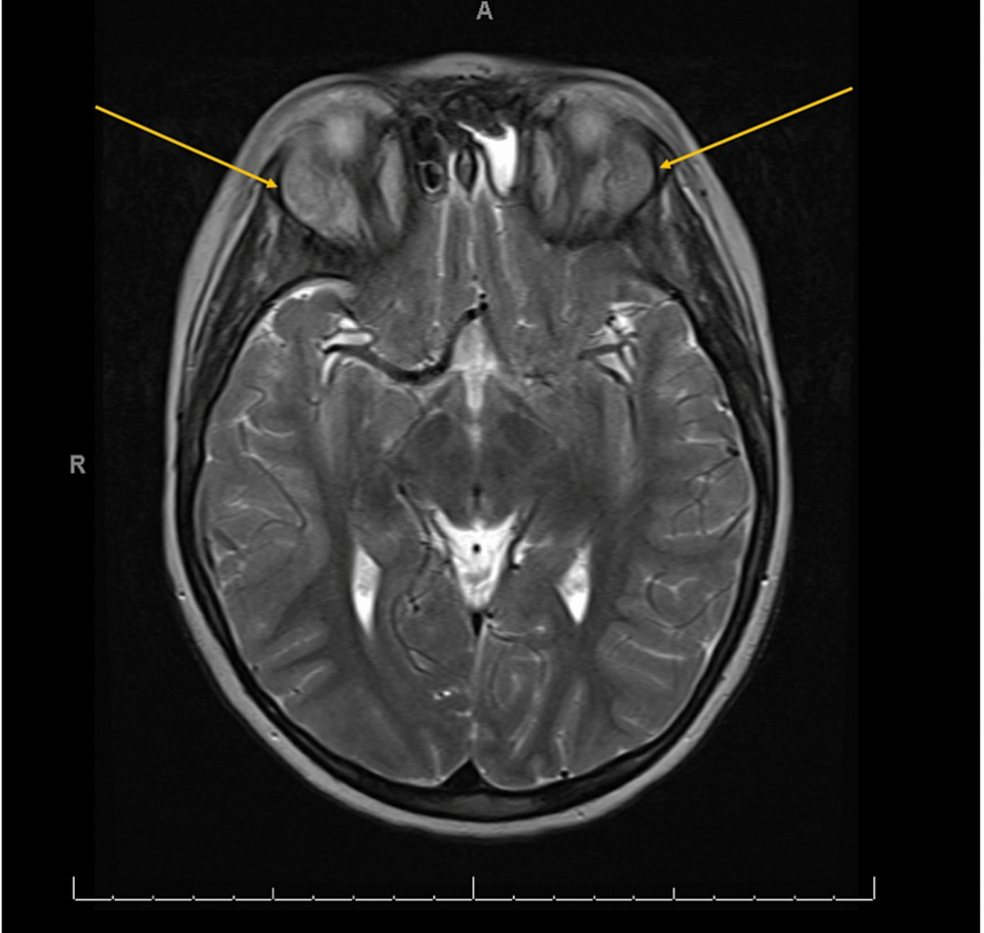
Axial T2-weighted MRI demonstrating intermediate to slightly low signal intensity of the orbital masses
